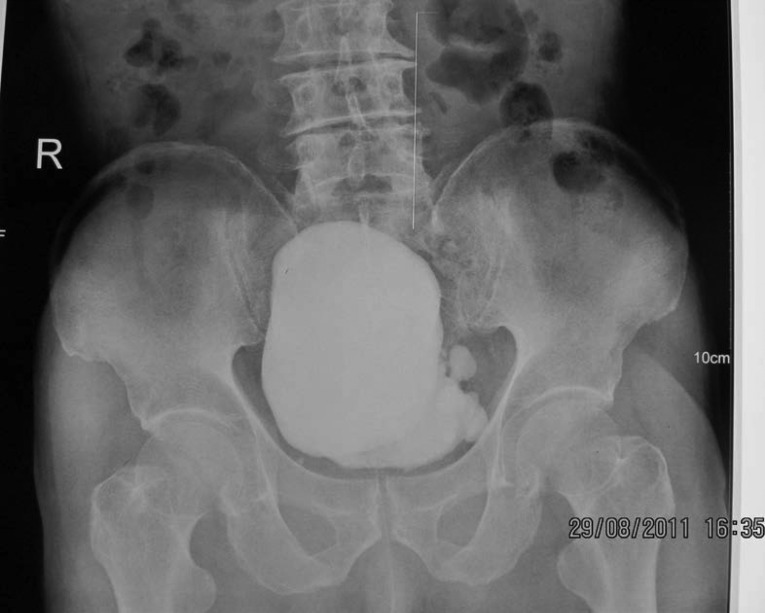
Preoperative radiography results of a patient (case 1) with bladder diverticulum accompanied by bladder calculus.
